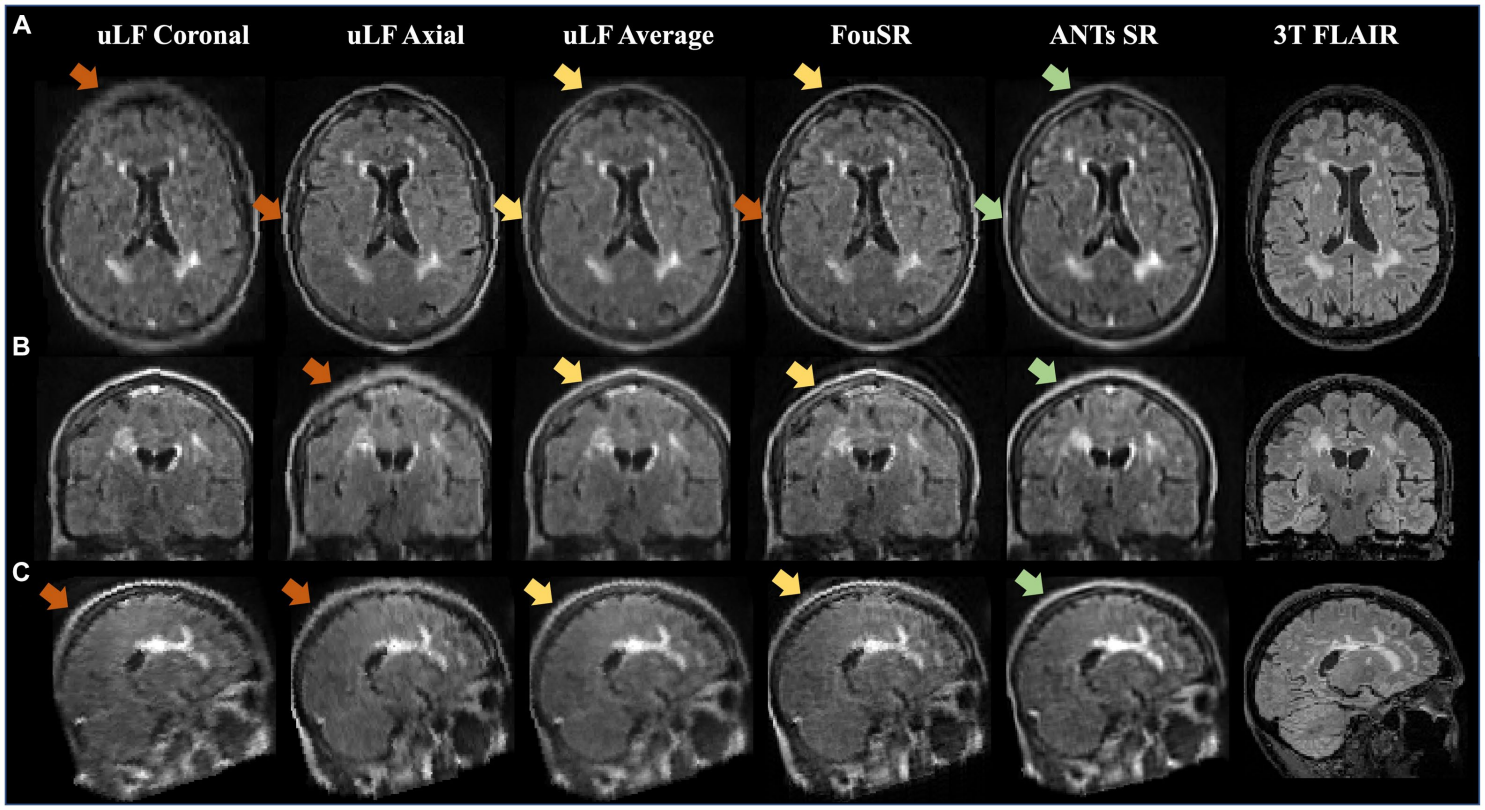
FouSR improves image quality across all three planes. Qualitative visualization of (A) axial, (B) coronal, and (C) sagittal slices in an MS case with high lesion burden in ULF FLAIR images, the average of ULF FLAIR images, after FouSR algorithm and after ANTs SR algorithm is shown. Arrows point to artifacts from partial voluming along the slice direction that is seen in the coronal and axial ULF (orange arrows) but reduced in FouSR and average images (yellow arrows) and absent in ANTs-SR images (green arrows).
